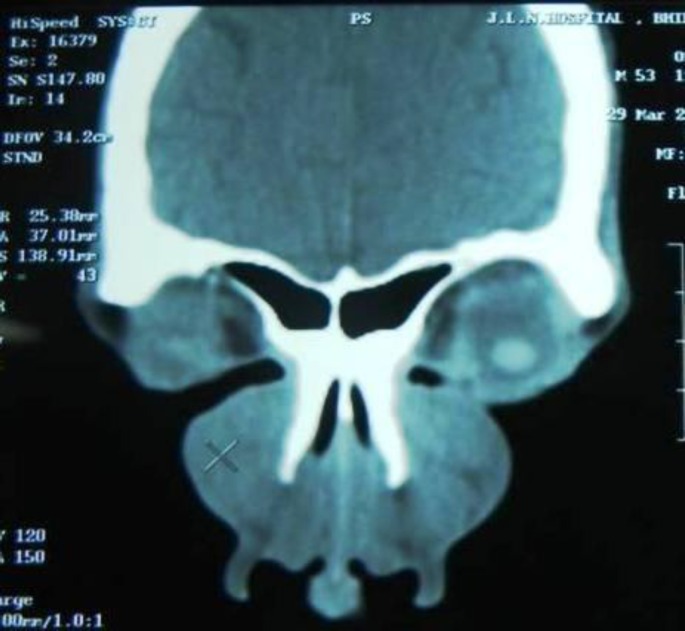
CT scan showing mild enhancing mass over nasal bones with no erosion of bones
